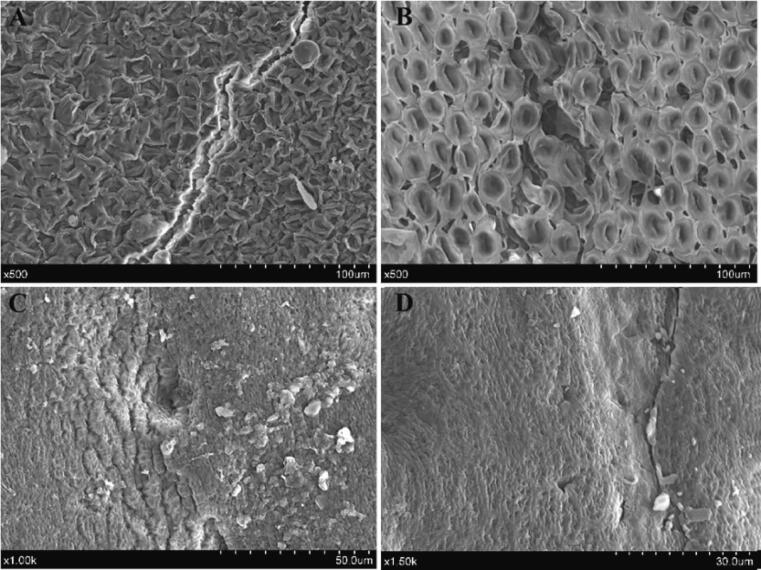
Microstructure of the soybean seed coat treated with dual-frequency ultrasound. Note: A: the surrounding surface of the hilum. B, C and D: the dorsal testa surface.
